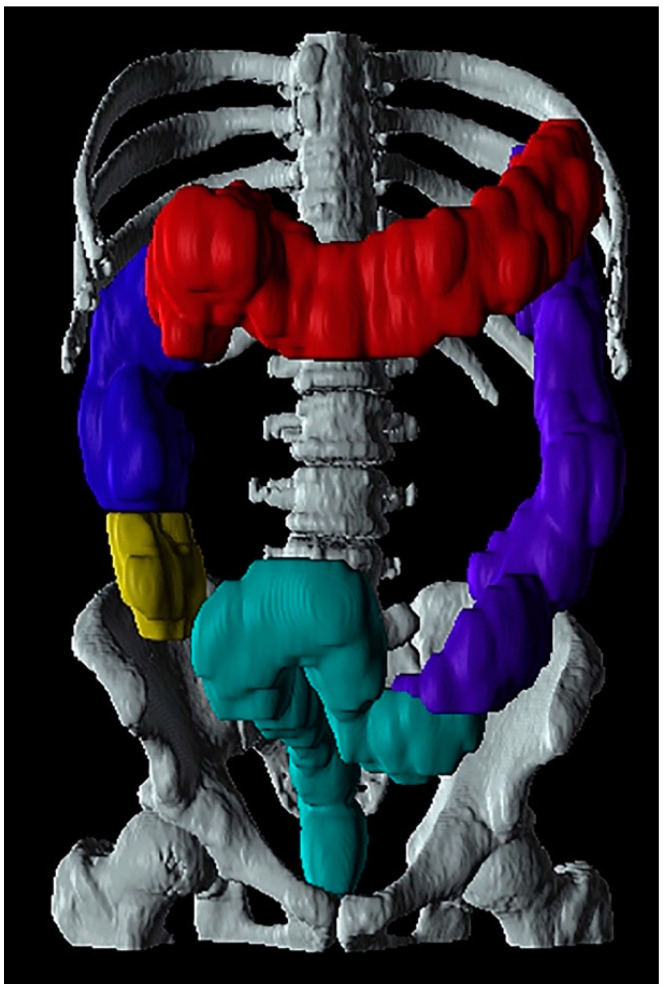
Example of colonic volumes from a computed tomography scan. Yellow: cecum, blue: ascending colon, red: transversal colon, purple: descending colon and turquoise: rectosigmoid colon. Used with permission from M. Klinge, Dissertation, January 2020.
